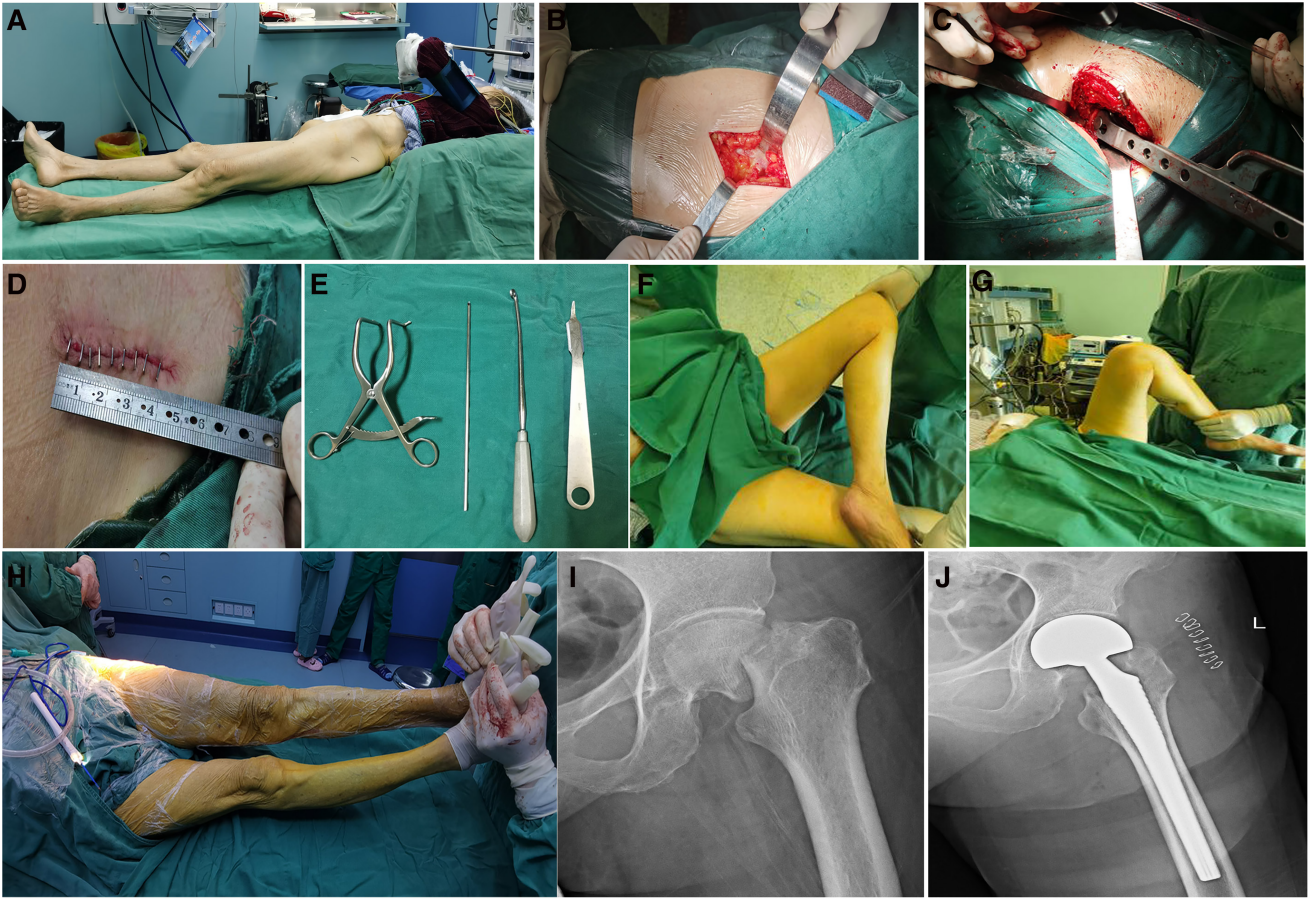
Representative case (87-year-old woman) of successful hemiarthroplasty using DSA. (A) Patient was placed in semi-recumbent position supine position on a surgical table. (B) A incision was made directly above the affected hip joint and superficial fascia was separated. (C) Femoral medullary cavity was grinded and reamed. (D) Special tools were required for the operation. (E) The length of the surgical incision was shown. (F,G) the movement of the hip joint after operation was inspected. (H) The lengths of lower limbs were compared afterward installing prosthesis. The x-ray images at preoperation (I) and postoperation (J).
